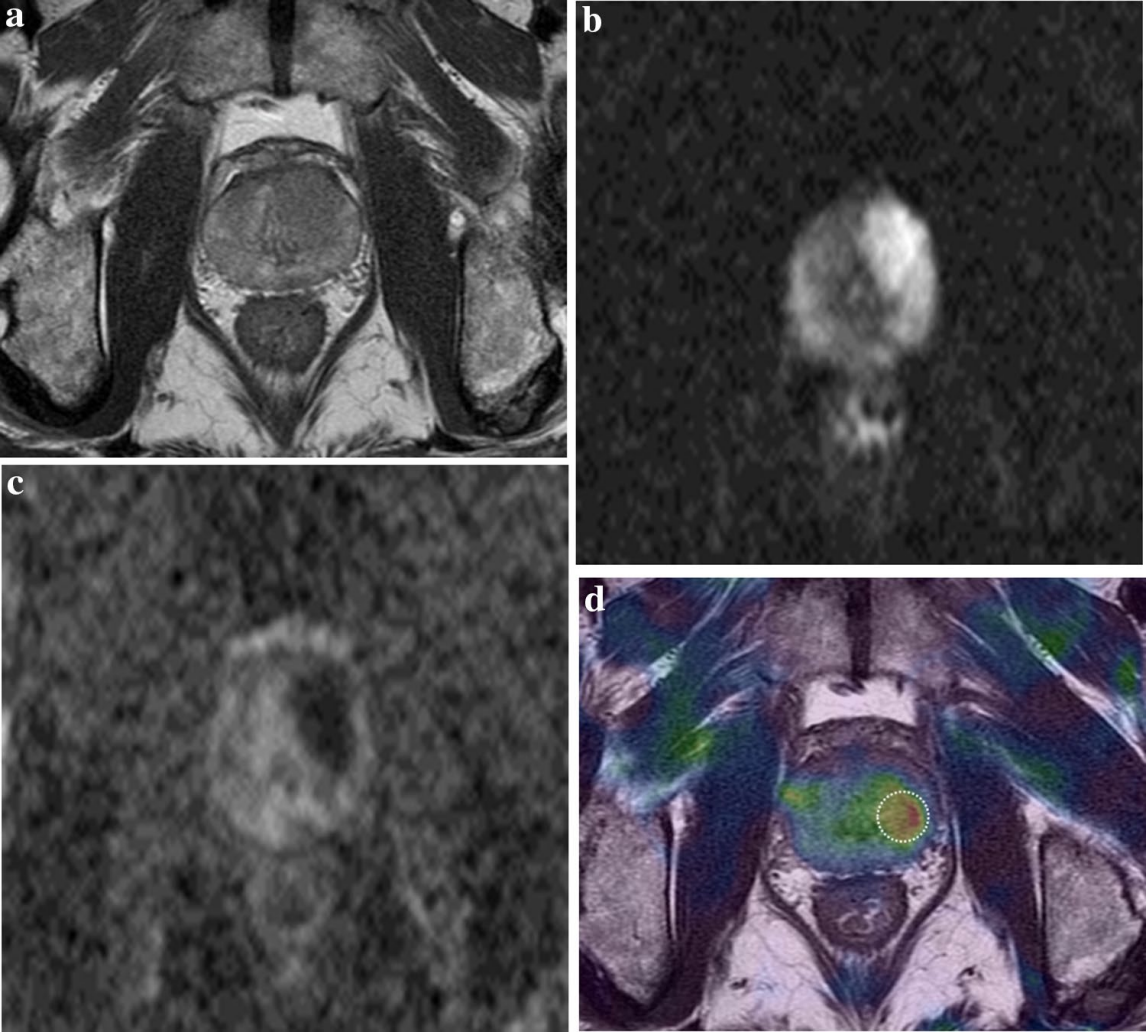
A 69-year-old man with prostate cancer confirmed after radical prostatectomy. (a) T2-weighted axial MR image showing a low-signal intensity lesion in the left apical transitional zone of the prostate. (b, c) Diffusion-weighted image (b = 1500 s/mm^2^) and ADC map show diffusion restriction in the corresponding area (ADC value, 0.528 × 10^−3^ m^2^/s). (d) Elastogram shows that the mean stiffness of the tumor is 6.218 kPa.
